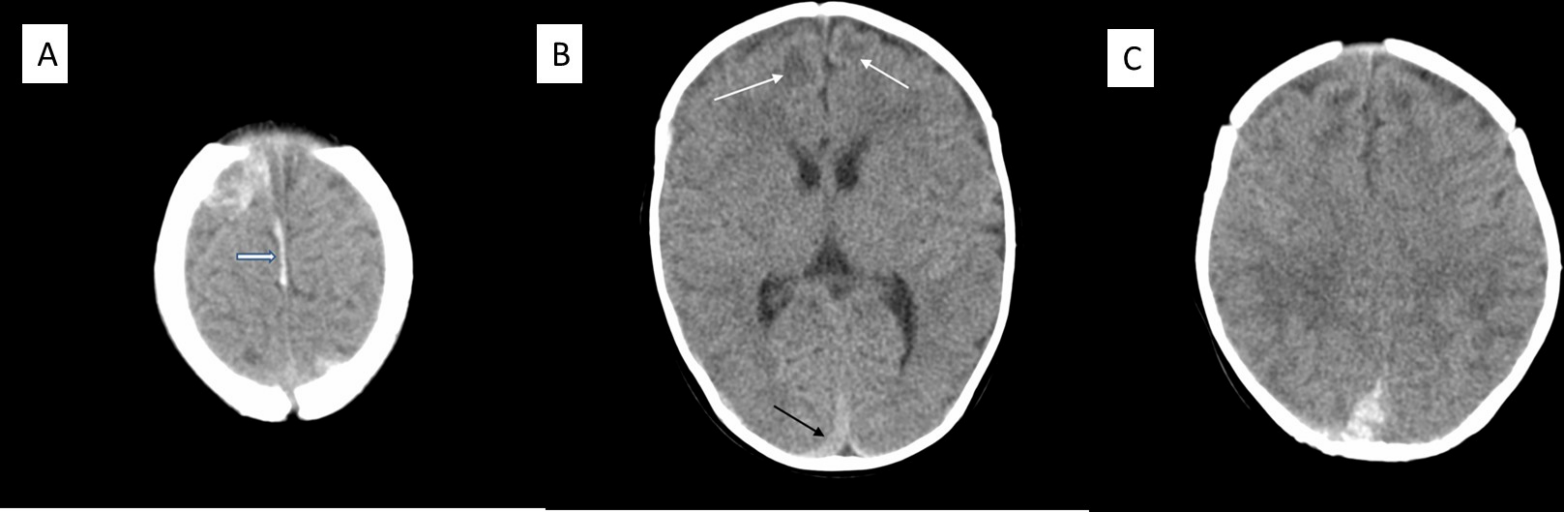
CT images of cases 8. There is acute subarachnoid blood in bilateral sulci over the vertex (A) and suspected cortical venous thromboses (arrow on one of them in A). There is a thin chronic subdural hematoma in the right frontotemporal region, and a localized fresh subdural hematoma in the right parietal region (black arrow in B). There is a small amount of fresh blood to the right of the confluens sinuum (C), probably extraaxially. In addition, there are symmetrical low attenuating parenchymal changes of unspecific appearance (white arrows in B).
